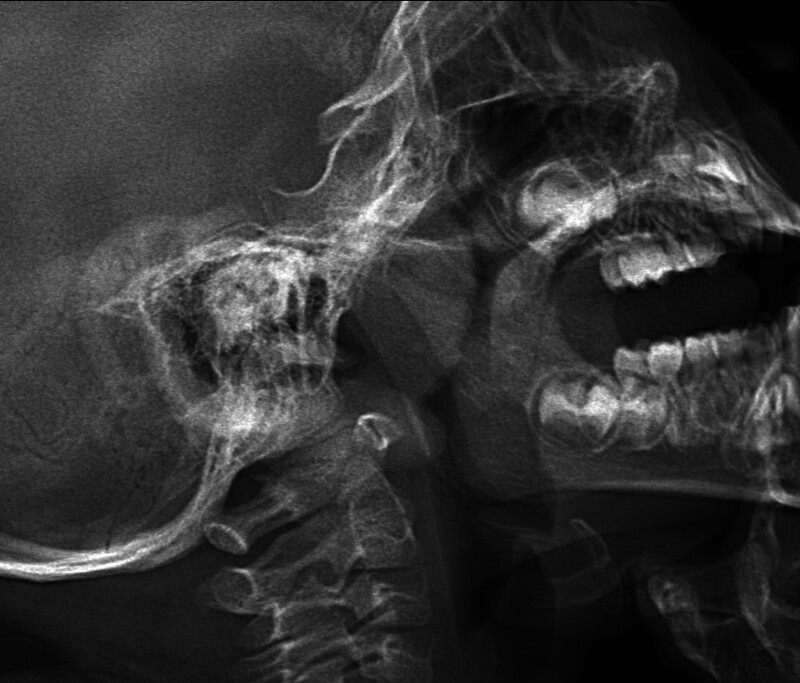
Neck X-ray: hypertrophy of the tonsils and adenoids.
